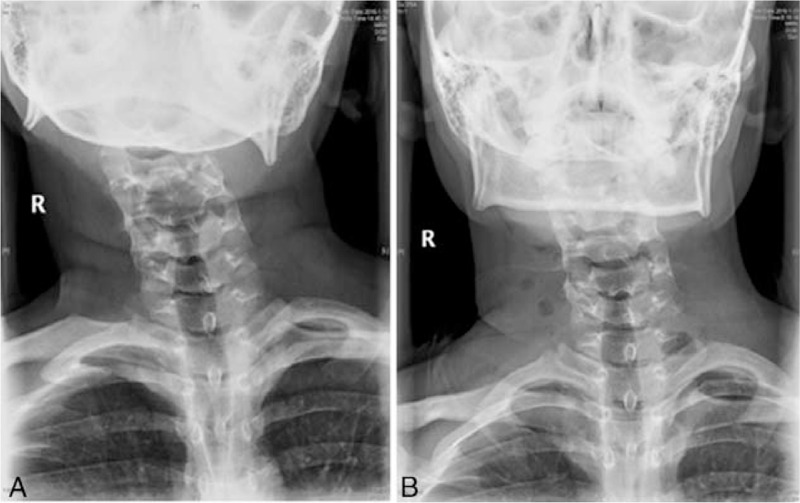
Fig. 4-A and Fig. 4-B show anteroposterior views of a patient's radiographs before and after surgery, respectively.
